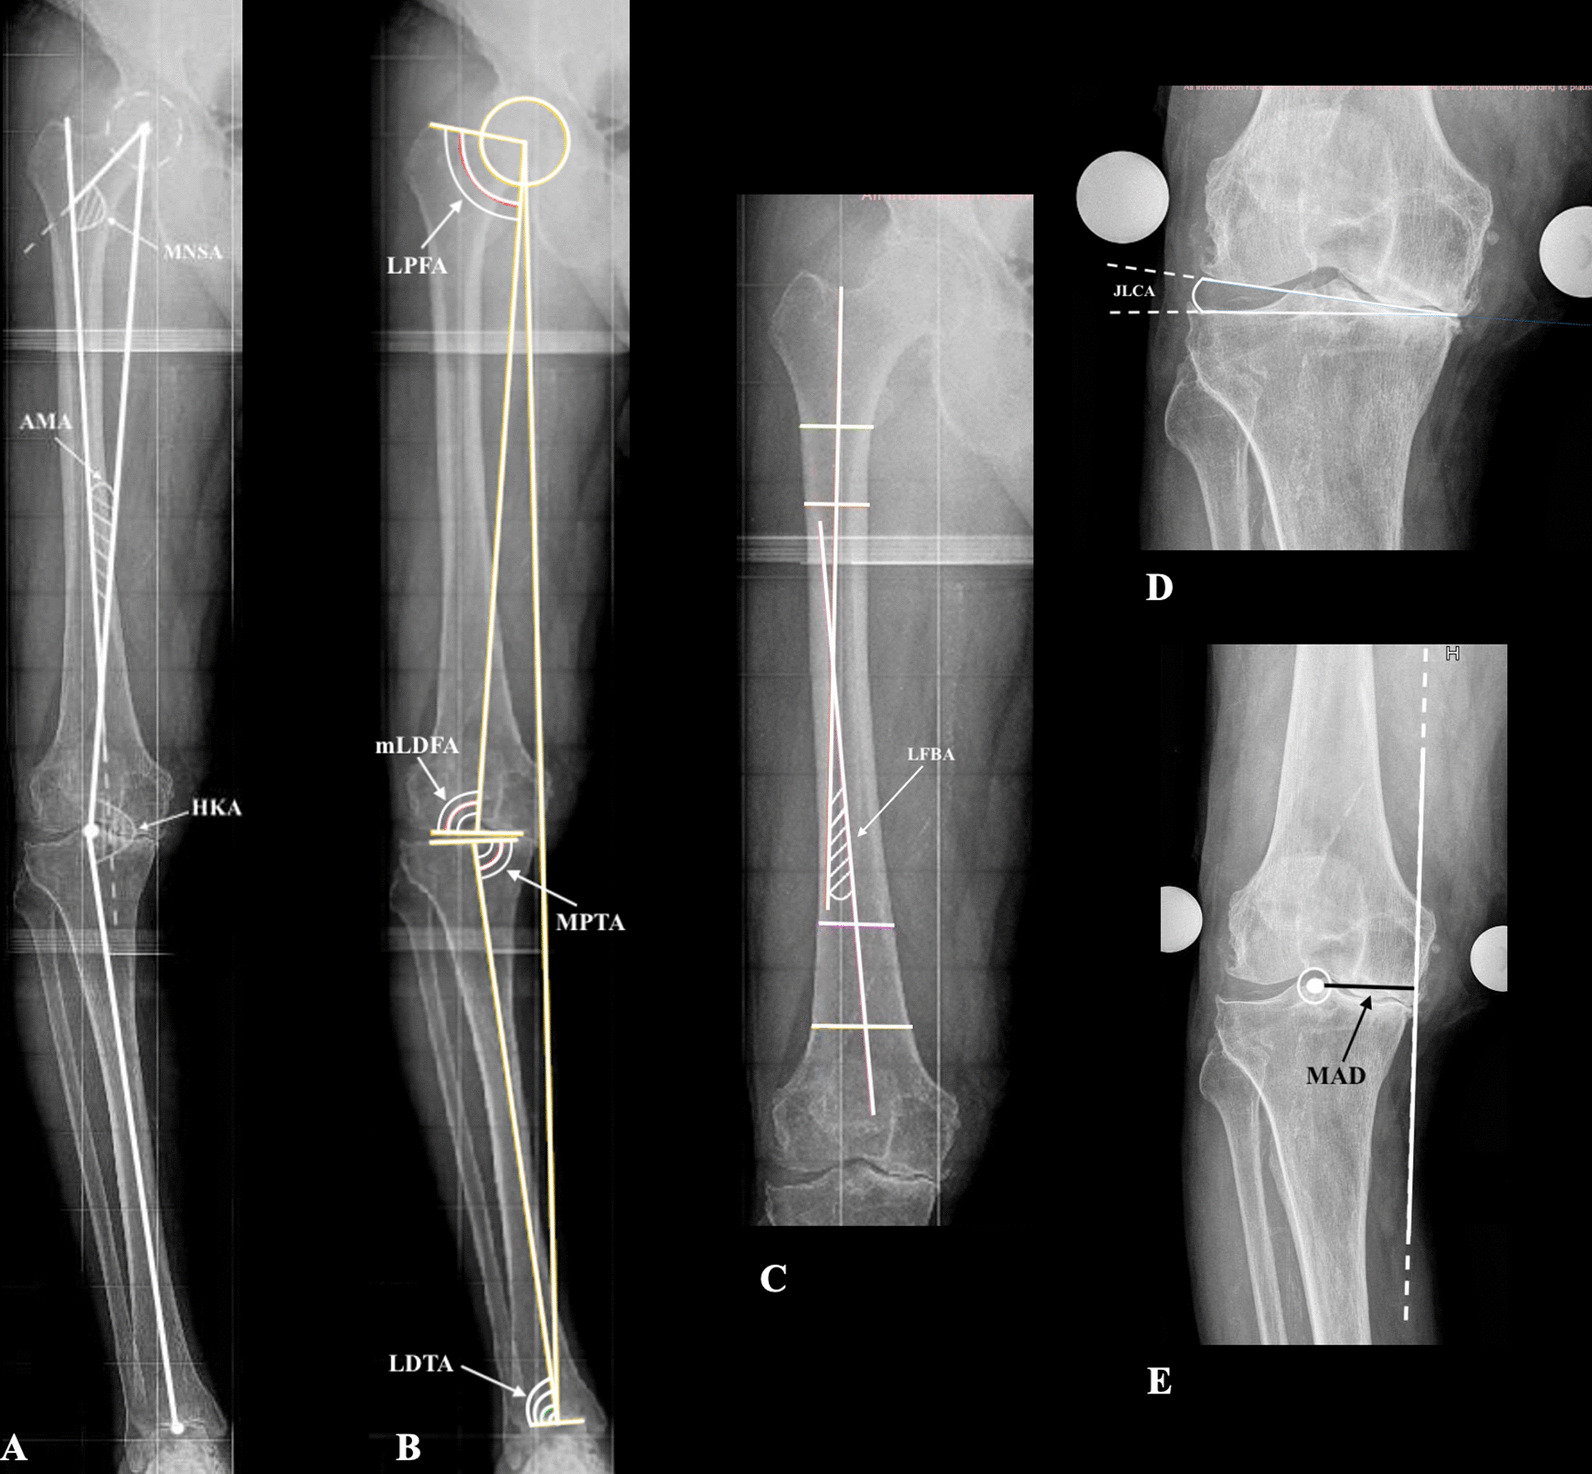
Radiographic assessment of preoperative measurements. A
